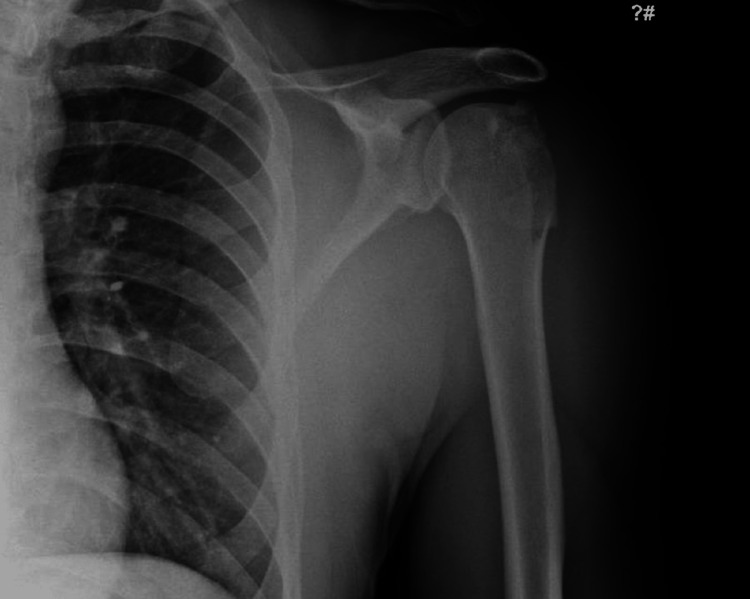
Post-reduction anteroposterior radiograph Demonstrating a successful reduction of the shoulder joint. Note that the greater tuberosity is not so well defined on this view.
